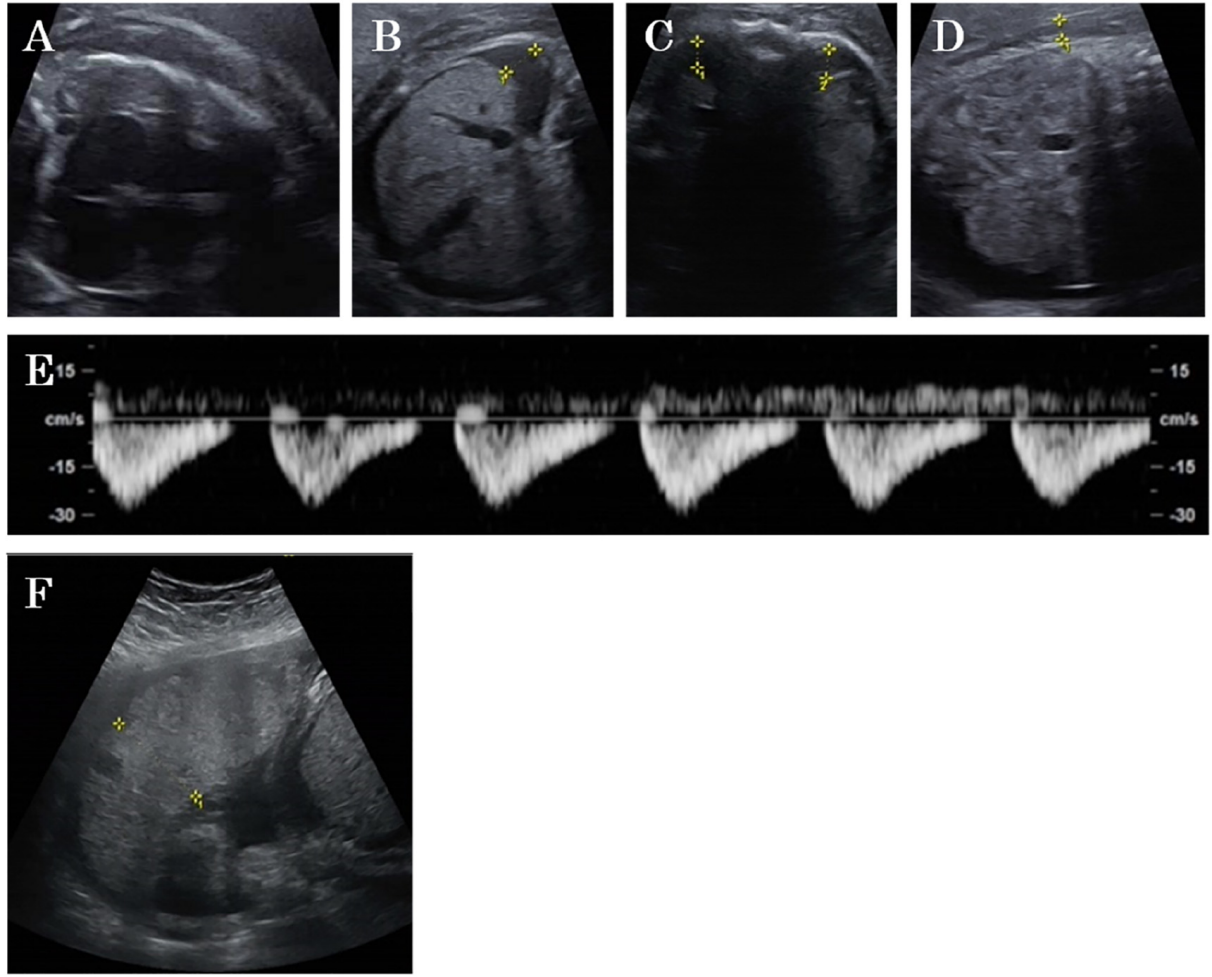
Transabdominal ultrasonography at the first visit. Fetus (tomography): cardiomegaly (A), ascites (B), bilateral pleural effusions (C), and subcutaneous edema (6.4 mm) (D). Umbilical artery (color Doppler methods): absent umbilical artery end-diastolic flow (E) Placenta (tomography): The placenta was not thickened (46 mm), the internal echogenicity was uniform (F), and there was no mass lesion.
